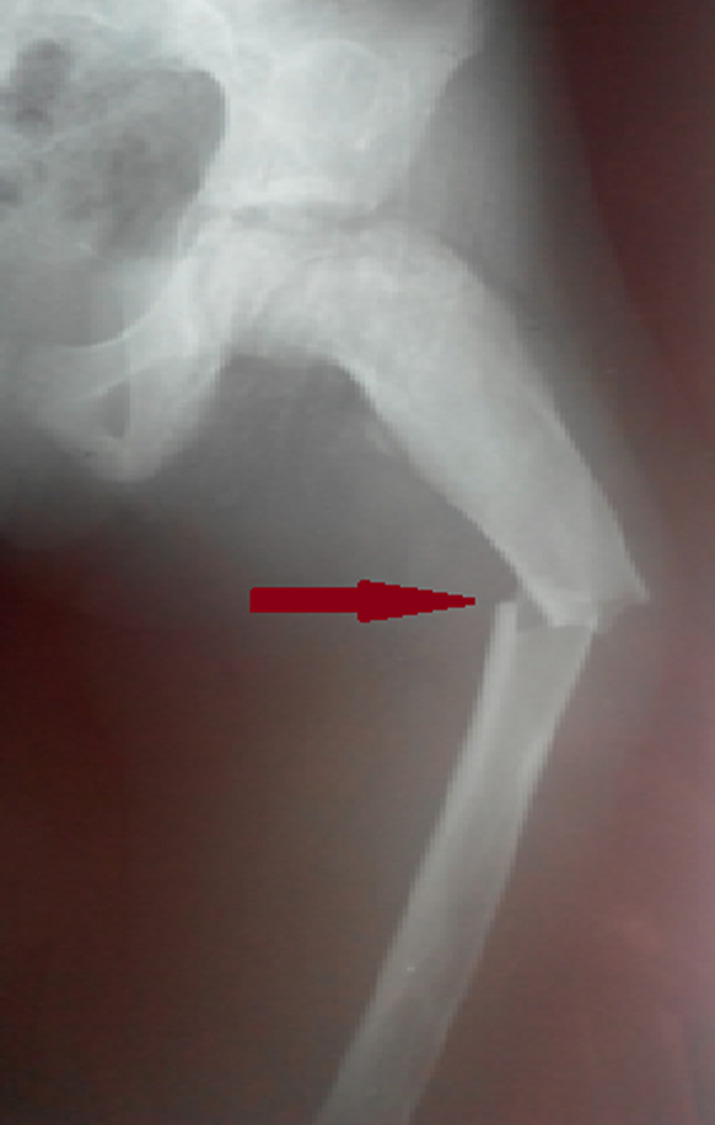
radiographie standard objectivant la fracture sous trochantérienne du fémur
